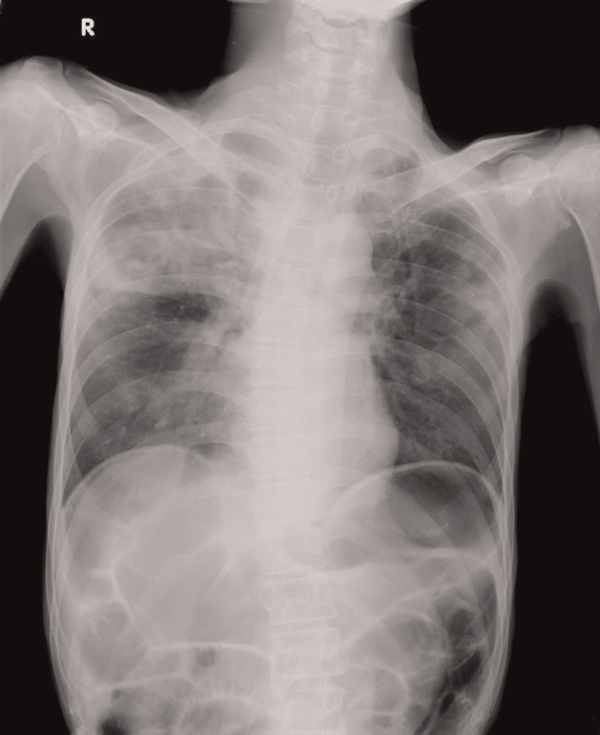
Chest radiograph at initial showing consolidation in bilateral upper lobes of the lung with multiple cavities and non-cavitary disease in the right middle lobe.
